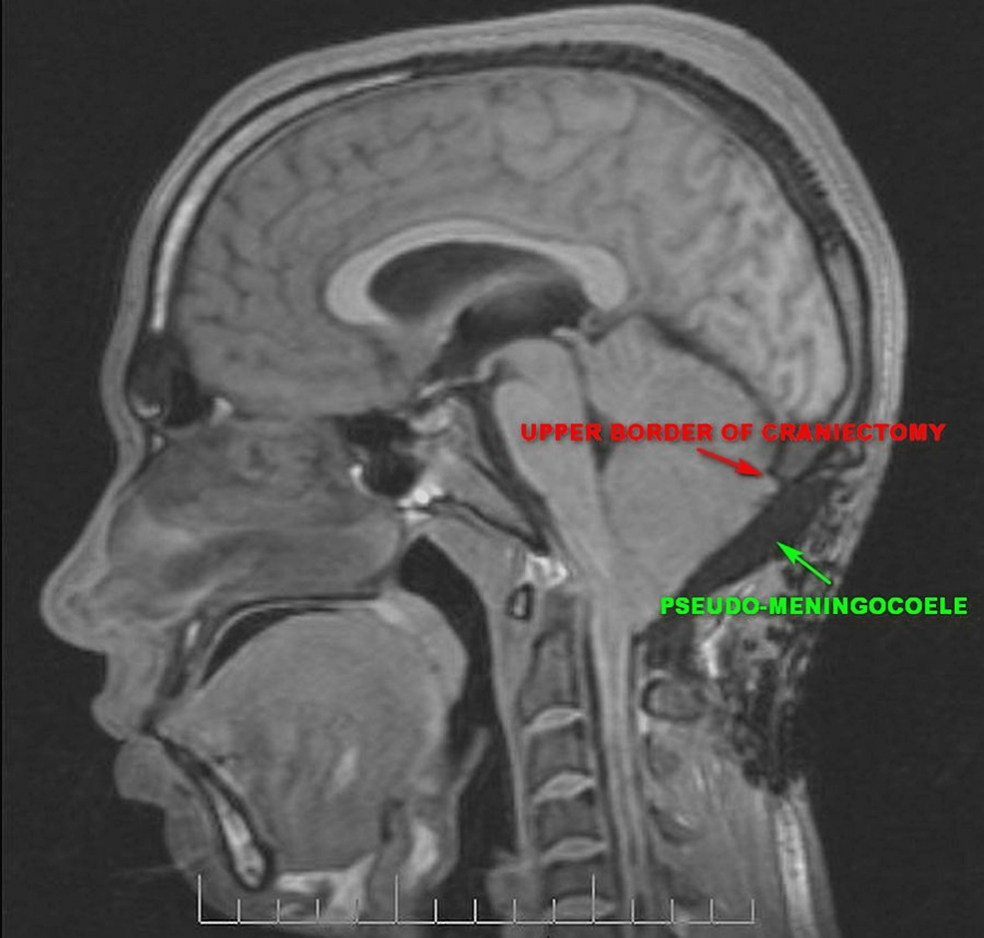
T1 T1 weighted sagittal MRI after the first surgery showing the extent of bone removal, the disappearance of the cervicothoracic syrinx, and the widening of the prepontine cistern. MRI: magnetic resonance imaging
